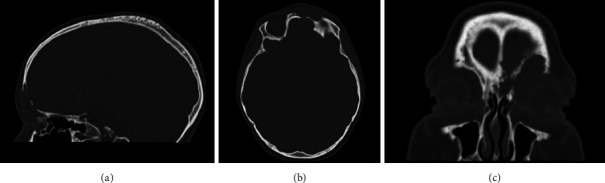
Pretreatment maxillofacial CT scan ((a) sagittal view, (b) axial view, and (c) coronal view) showing a resorption of the frontal bone wall.
